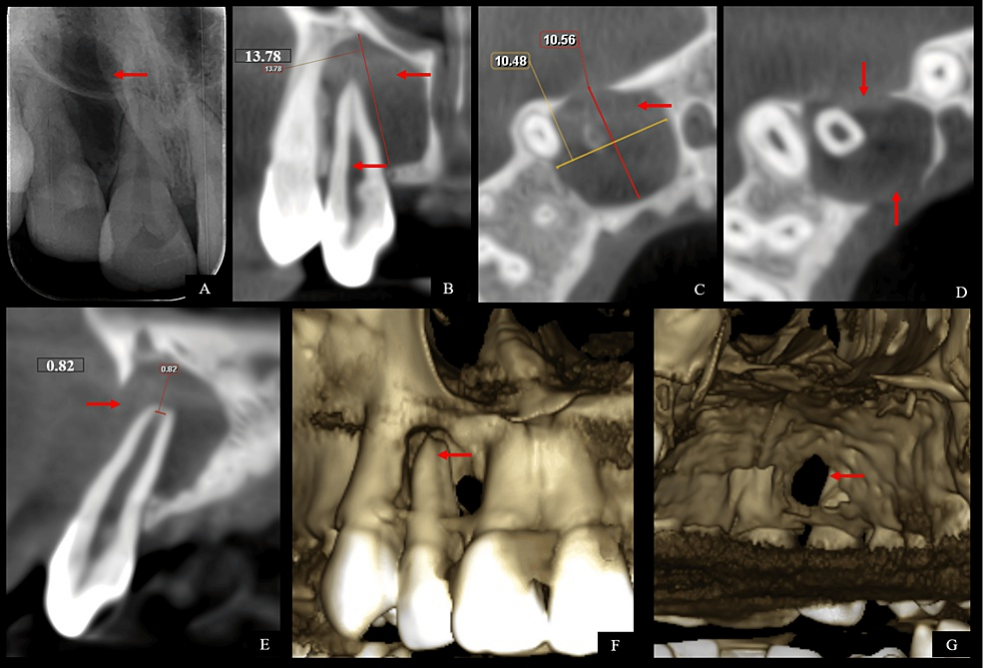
Pre-operative radiographic images (A) Intra-oral periapical radiograph with 12 showing a large periapical radiolucent shadow, (B) coronal cone-beam computed tomographic view showing wide canal and a radiolucent shadow with well-corticated borders measuring 13.78 mm superior-inferiorly and resorbed root apex with 12, (C) axial view showing the lesion measuring 10.48 mm mesiodistally and 10.56 mm buccopalatally, (D) axial view showing perforation of the labial and lingual cortical plate, (E) sagittal view of 12 showing root resorption with an apical diameter of 0.82 mm and loss of buccal cortical plate, (F) bony view showing a tunnel defect with 12, and (G) bony view showing a tunnel defect with 12.
